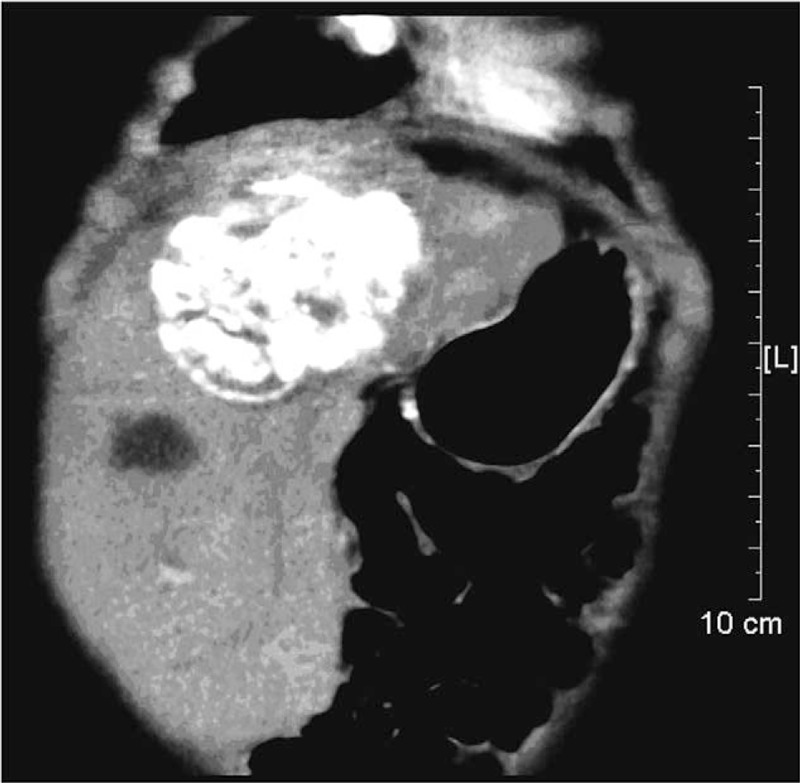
Contrast-enhanced CT scan showing a biloma with fluid collection. CT = computed tomography.
